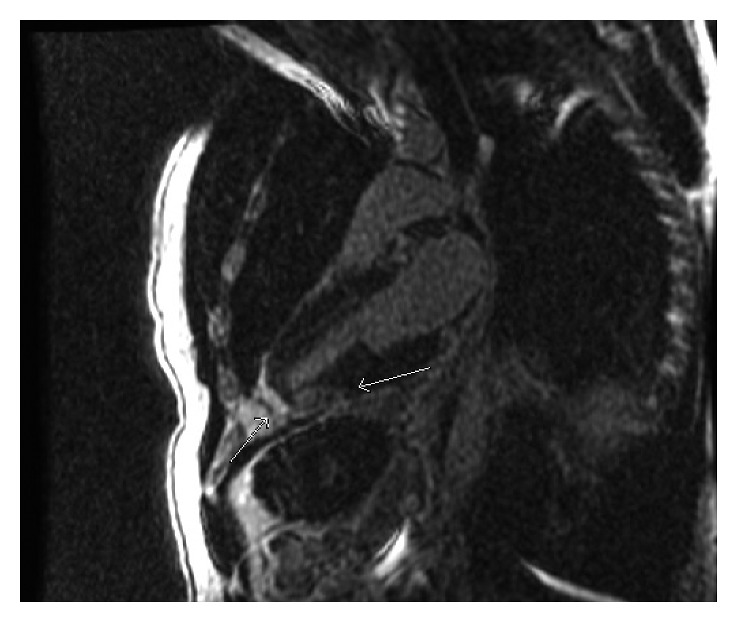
Delayed postcontrast cardiac MRI 2-chamber view demonstrates midmyocardial enhancement in the inferior wall of the left ventricle adjacent to the apex. The subendocardial region is sparred (right arrow). Mild pericardial delayed enhancement (left arrow).
